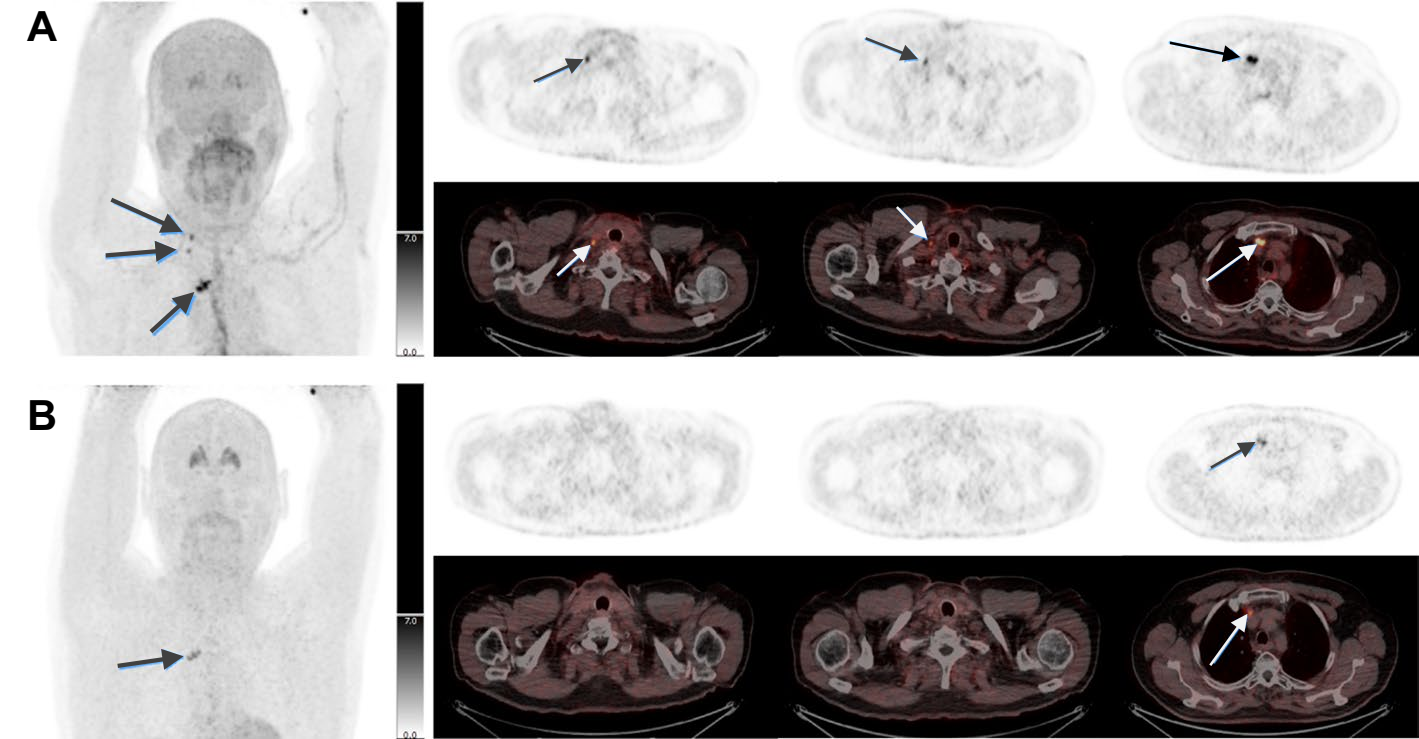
A 65-year-old man (patient 3). All lesions were verified by biopsies as true positive. A Imaging 15 min p.i. showed focal ^18^F-FDOPA uptake corresponding to two cervical lymph nodes (SUV_max_ = 6) and three thoracic lymph nodes (SUV_max_ = 10). B
